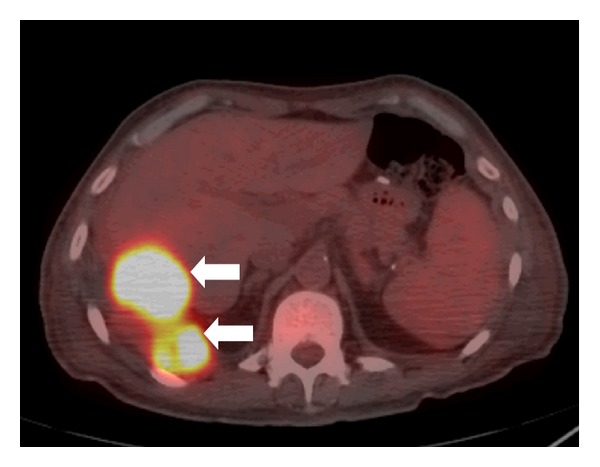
An axial chest positron emission tomography/computed tomography (PET/CT) scan showing two hypermetabolic fluorodeoxyglucose- (FDG-) avid mass lesions involving the right diaphragmatic pleura, suggestive of neoplastic lesions (white arrows).
